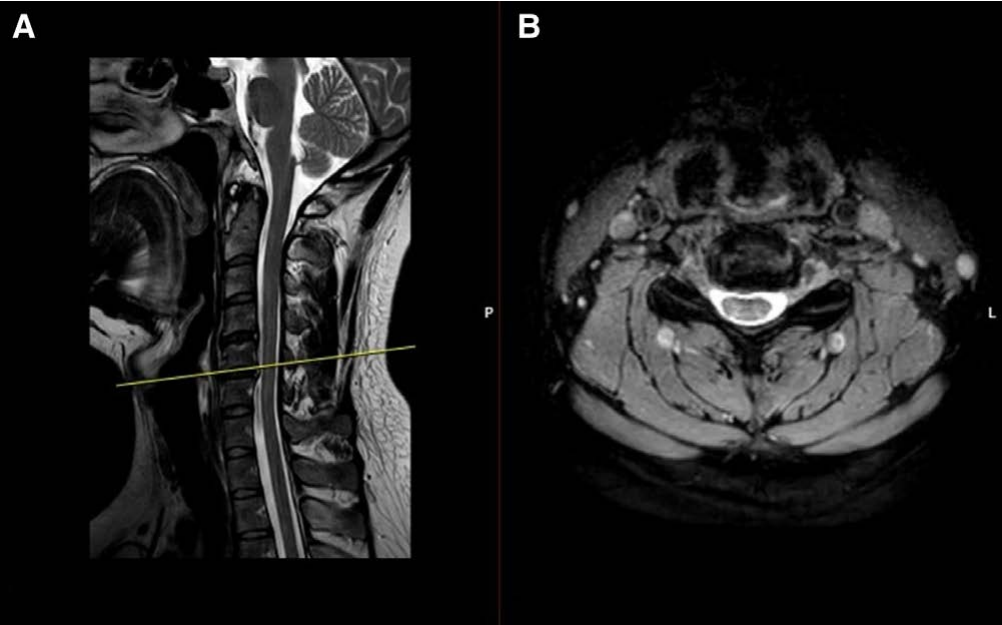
(A) Sagittal section of cervical MRI showing a broad-based posterior central-left paramedian mild protrusion at the C4–C5 level and a broad-based posterior central protrusion at the C5–C6 level. (B) Transverse section of cervical MRI at the C5–C6 level showing narrowing of the right neural foramen due to disc protrusion with ventral root compression, while the left neural foramen remains open with no root compression. MRI = magnetic resonance imaging.
